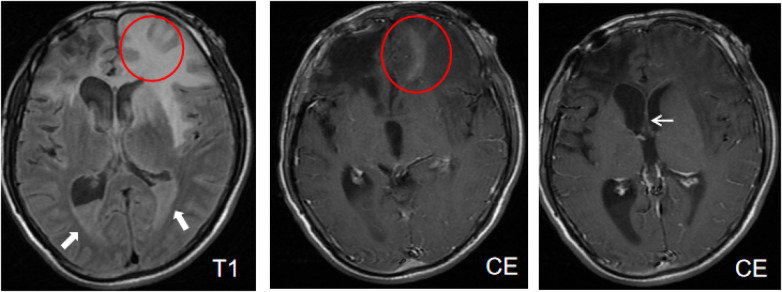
Cranial MRI: rupture of the brain abscess is visualized on T1-weighted and contrast-enhanced (CE) sequences, with only the abscess wall remaining (red circle); empyema is present in the posterior horns of the bilateral ventricles (thick arrow); the midline shift is significantly reduced (thin arrow).
